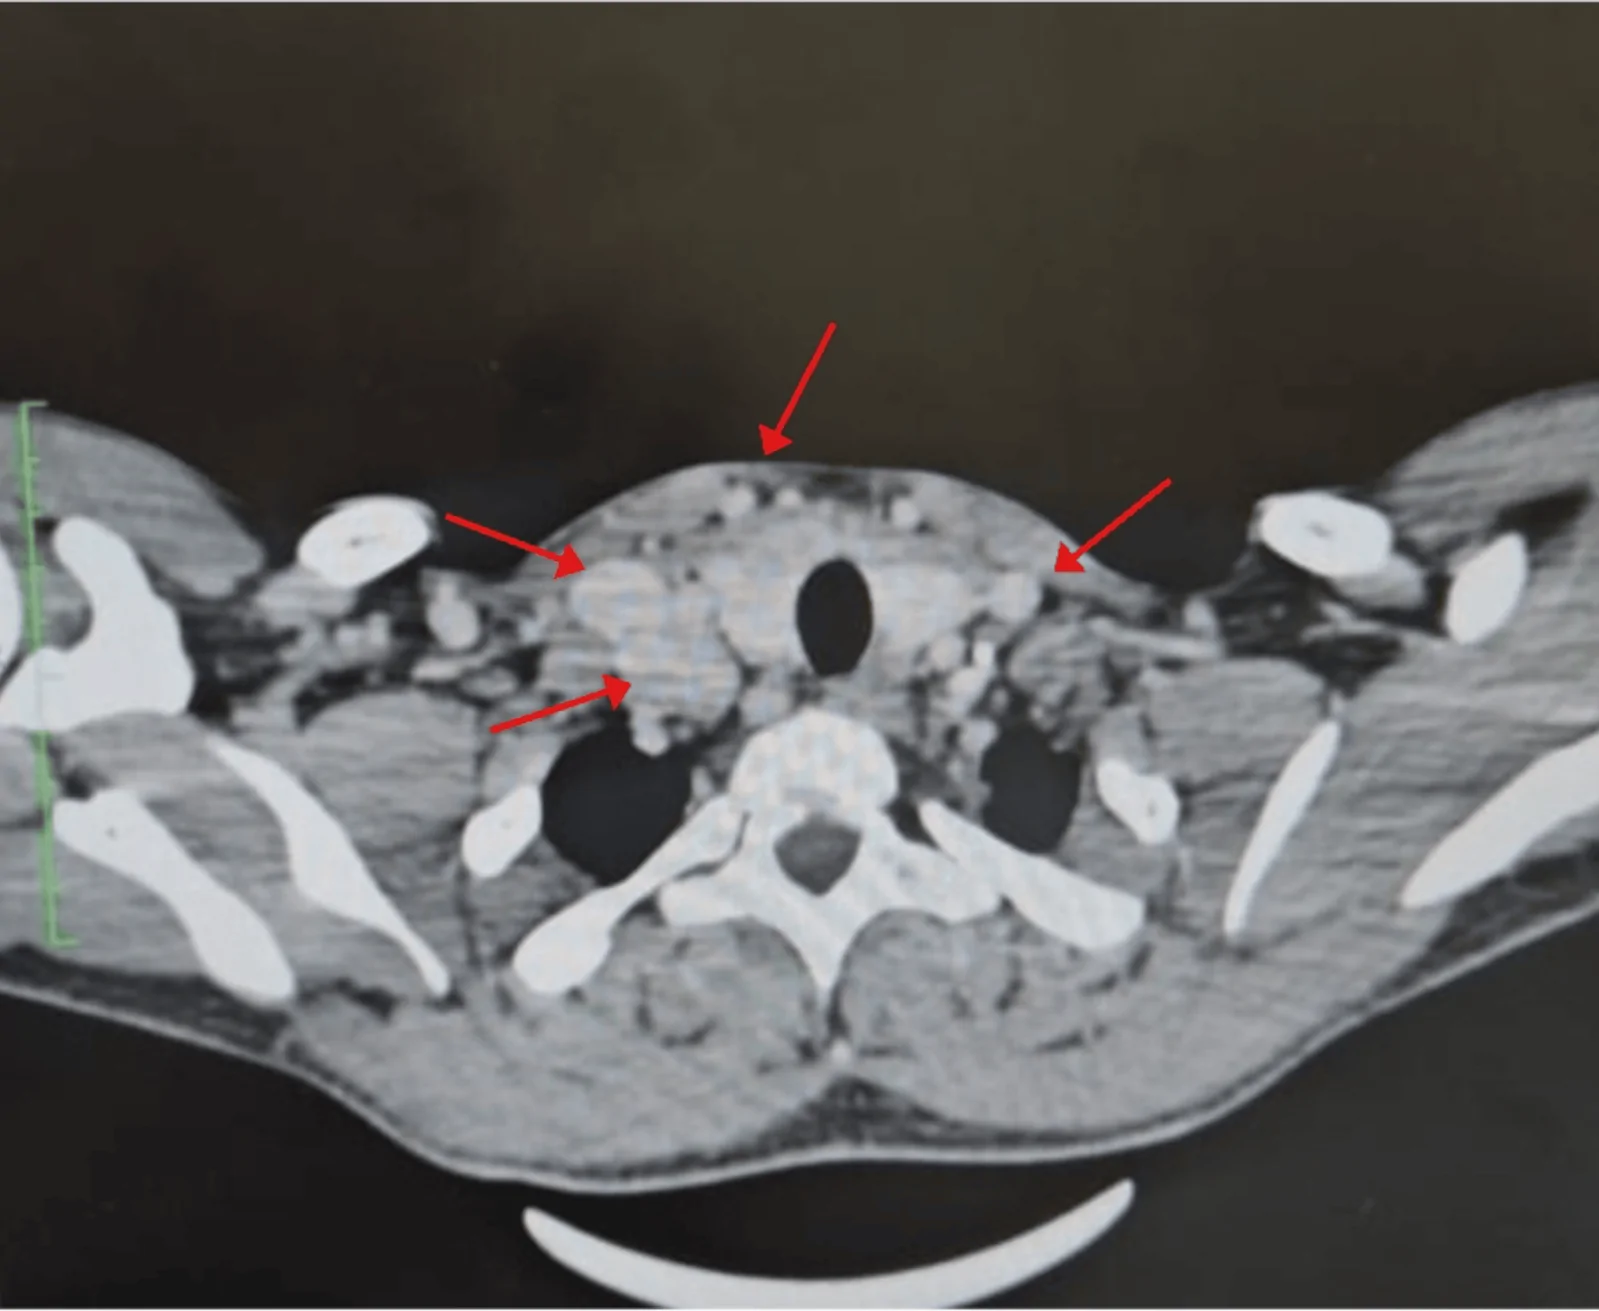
Axial contrast-enhanced cervicothoracic computed tomography (CT) at the level of the thoracic inlet Massive involvement of the central compartment is revealed, with multiple dense prevascular and pretracheal lymphadenopathies that mimic the structural radiological pattern of a primary mediastinal lymphoma or germ cell tumor.
